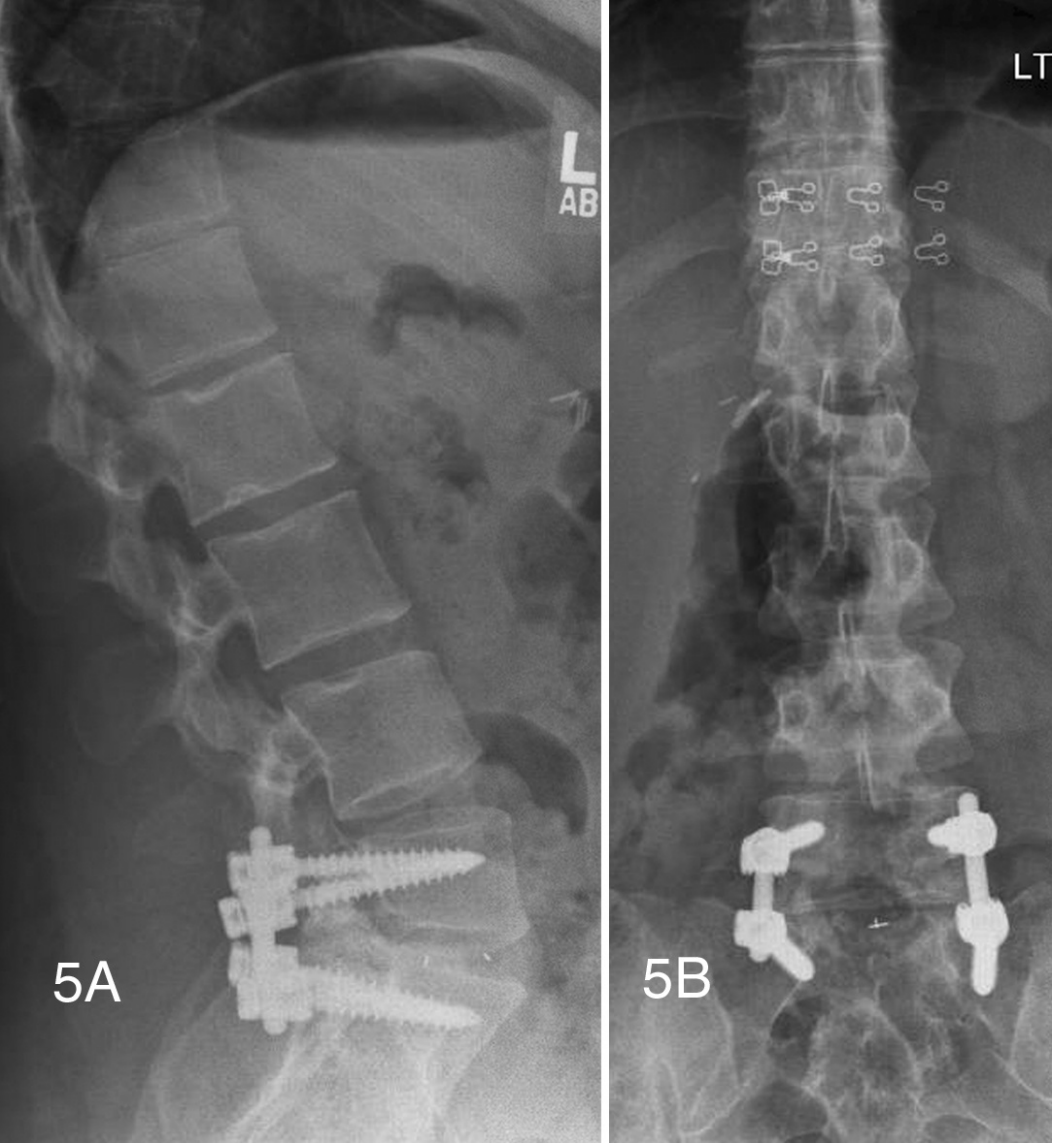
Postoperative lateral and AP radiographs (Figures 5A, 5B) Lateral and AP radiographs showing proper pedicle screw and oblique PLIF cage placement using TLIF technique.
